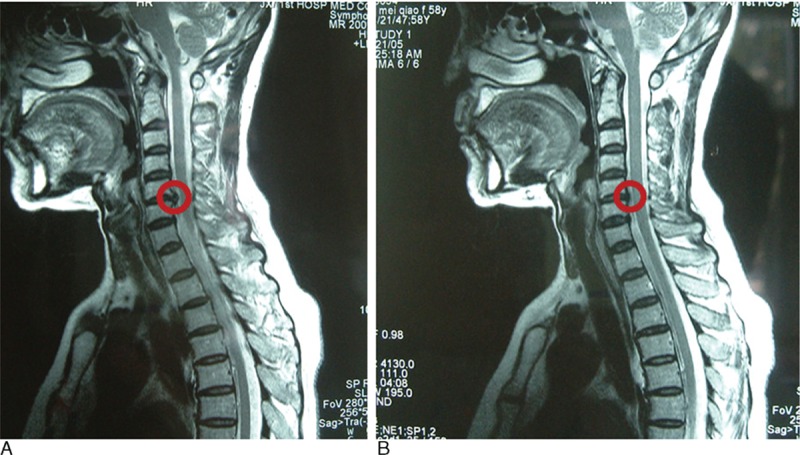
(A) Protrusion of the C5–6 disc demonstrated via a preoperative magnetic resonance imaging (MRI) scan. (B) Reduced protrusion of the C5–6 disc demonstrated via a postoperative MRI scan.
